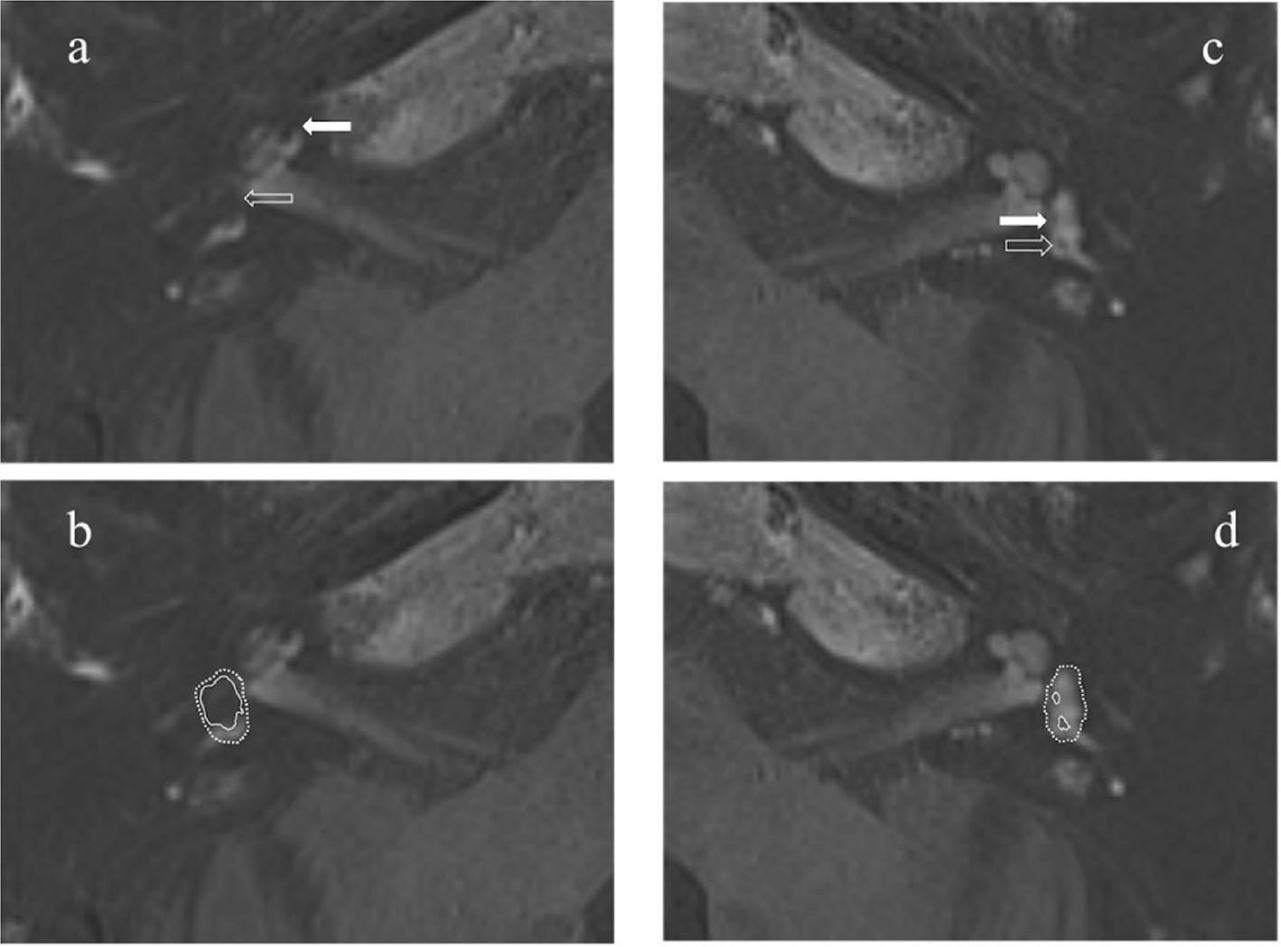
Delayed post-gadolinium 3D FLAIR axial images demonstrate (a) Severe vestibulo-cochlear endolymphatic hydrops. Severe cochlear hydrops (solid arrow) and severe vestibular hydrops (open arrow) is indicated by the arrows. b The same image demonstrating severe vestibulo-cochlear hydrops with contouring of the vestibular endolymphatic and perilymphatic spaces. The vestibular endolymphatic space comprises the confluent saccule and utricle (central solid contour). This almost replaces the surrounding enhancing vestibular perilymphatic space (peripheral dotted contour) (c) Normal endolymphatic appearances. The normal cochlear duct is not perceptible whilst the normal sized non-enhancing saccule (solid arrow) and utricle (open arrow) are clearly visualised within the enhancing perilymphatic space (d) The same image demonstrating the normal endolymphatic spaces with contouring of the non-enhancing vestibular endolymphatic space comprising the saccule and utricle (central solid contour). It is surrounded by the enhancing vestibular perilymphatic space which is more clearly visualised (peripheral dotted contour)
